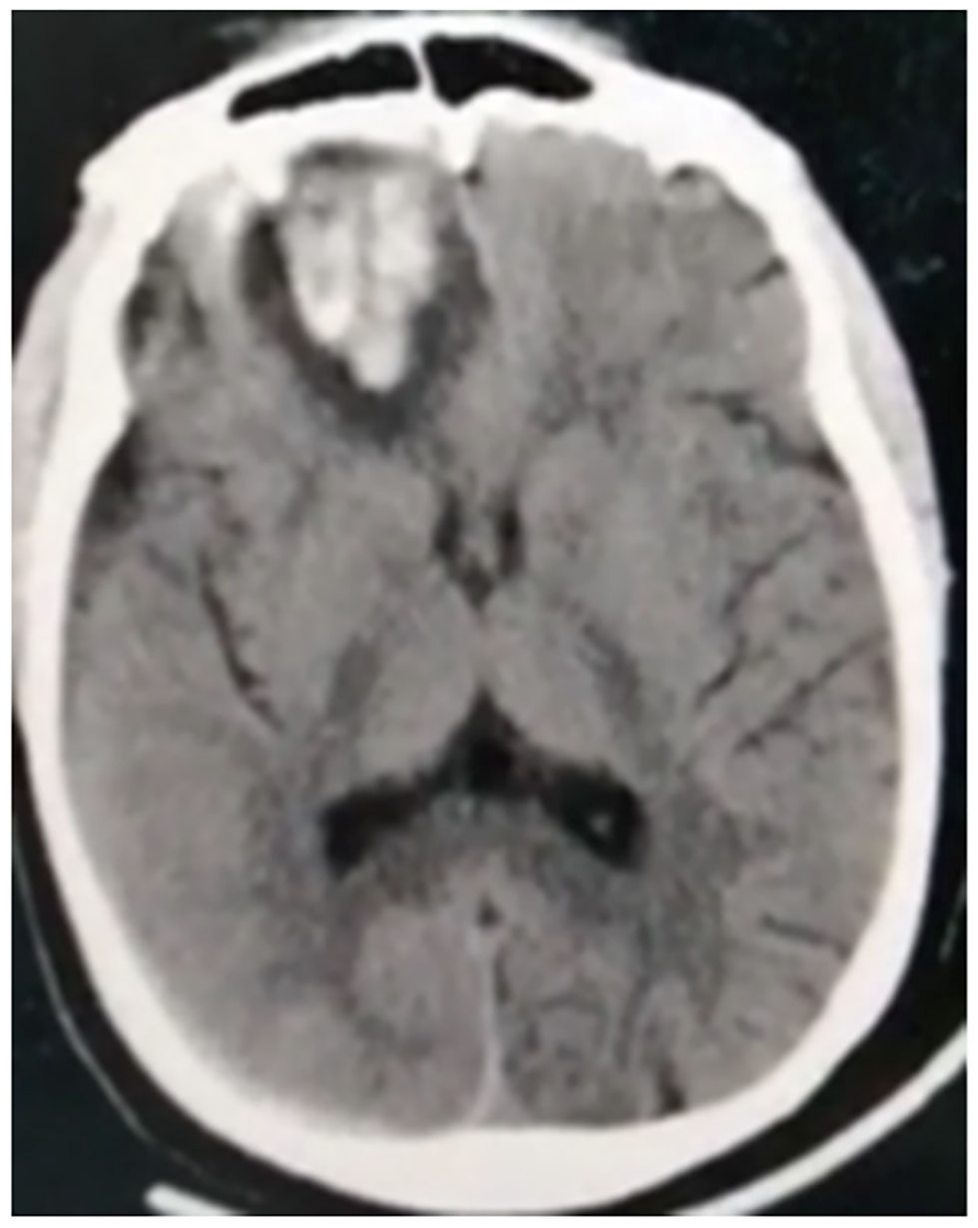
Non-contrast CT of a 38-year-old male patient shows intra-parenchymal right inferior frontal hematoma.
